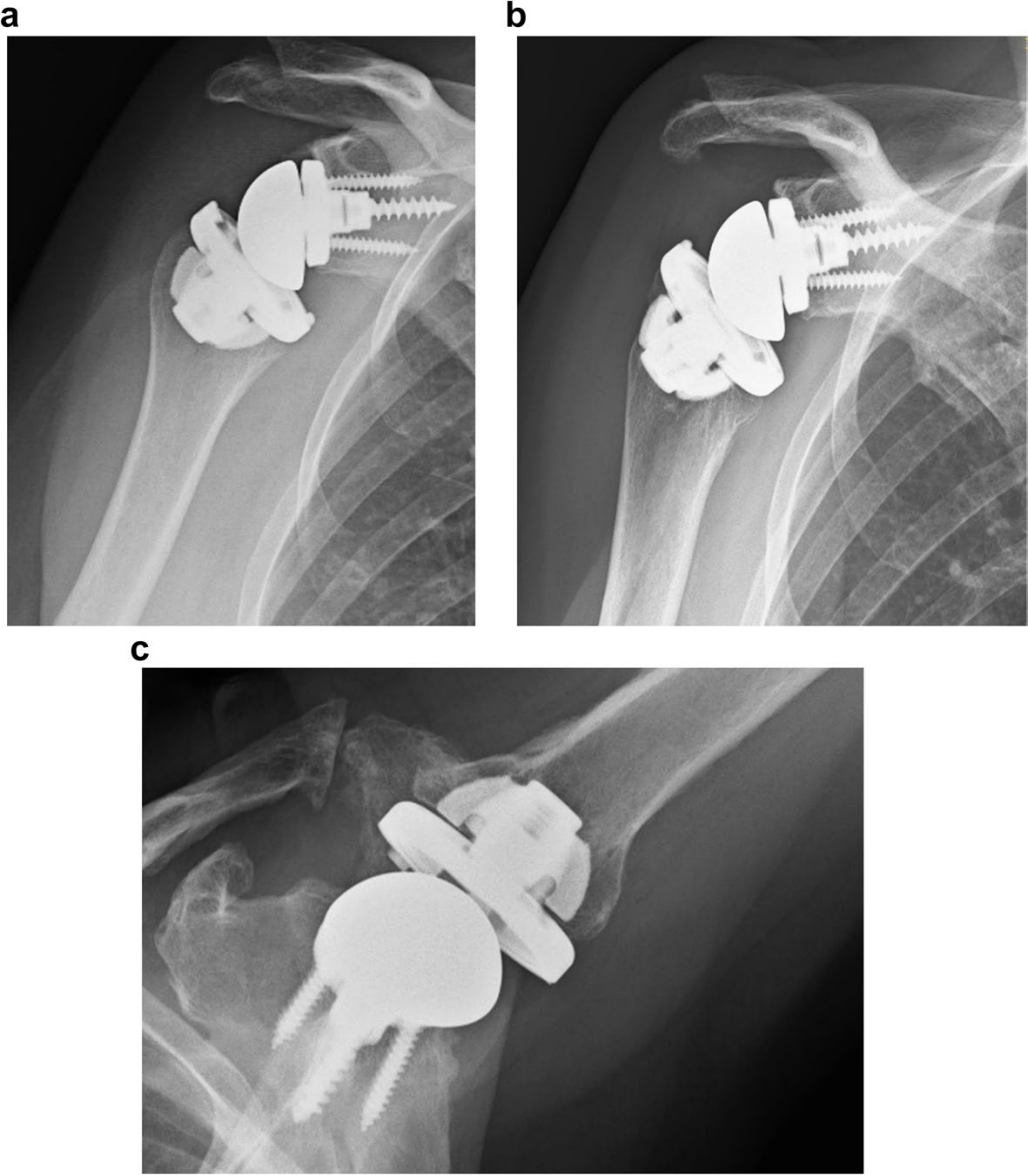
X-ray imaging postoperatively (a) and at follow-up (b/c) after 119 months showing significant resorption at the greater tuberosity of this 90-year-old woman at follow-up
